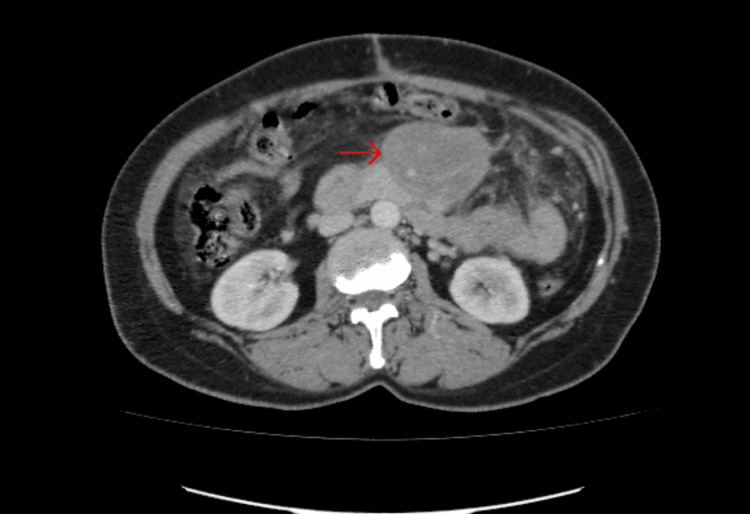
CT scan showing a mass encasing the mesenteric artery. A baseline CT scan shows a large mass. Red arrow pointing to the mass.
